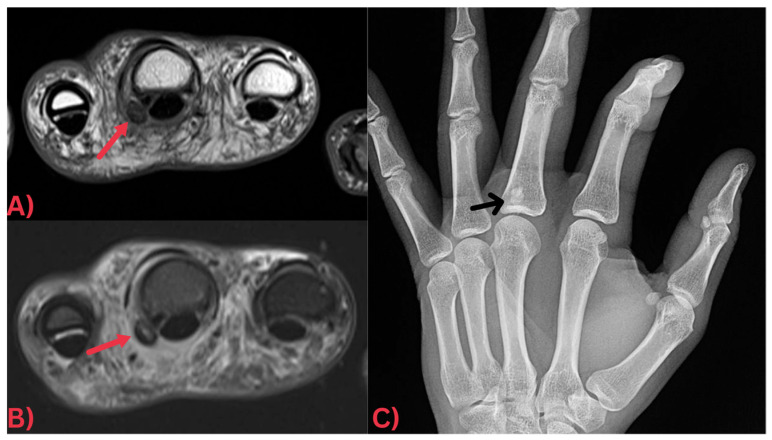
(A) Axial PD and (B) axial T2 fat-saturated MRI images of the hand demonstrating a well-defined globular low signal intensity focus at the volar capsule of the metacarpophalangeal joint of the middle finger (red arrows) with significant surrounding soft tissue edema. Note how this focus is more easily discernable on the (C) X-ray image of the hand, which clearly demonstrates that it is a focus of calcification (black arrow).
